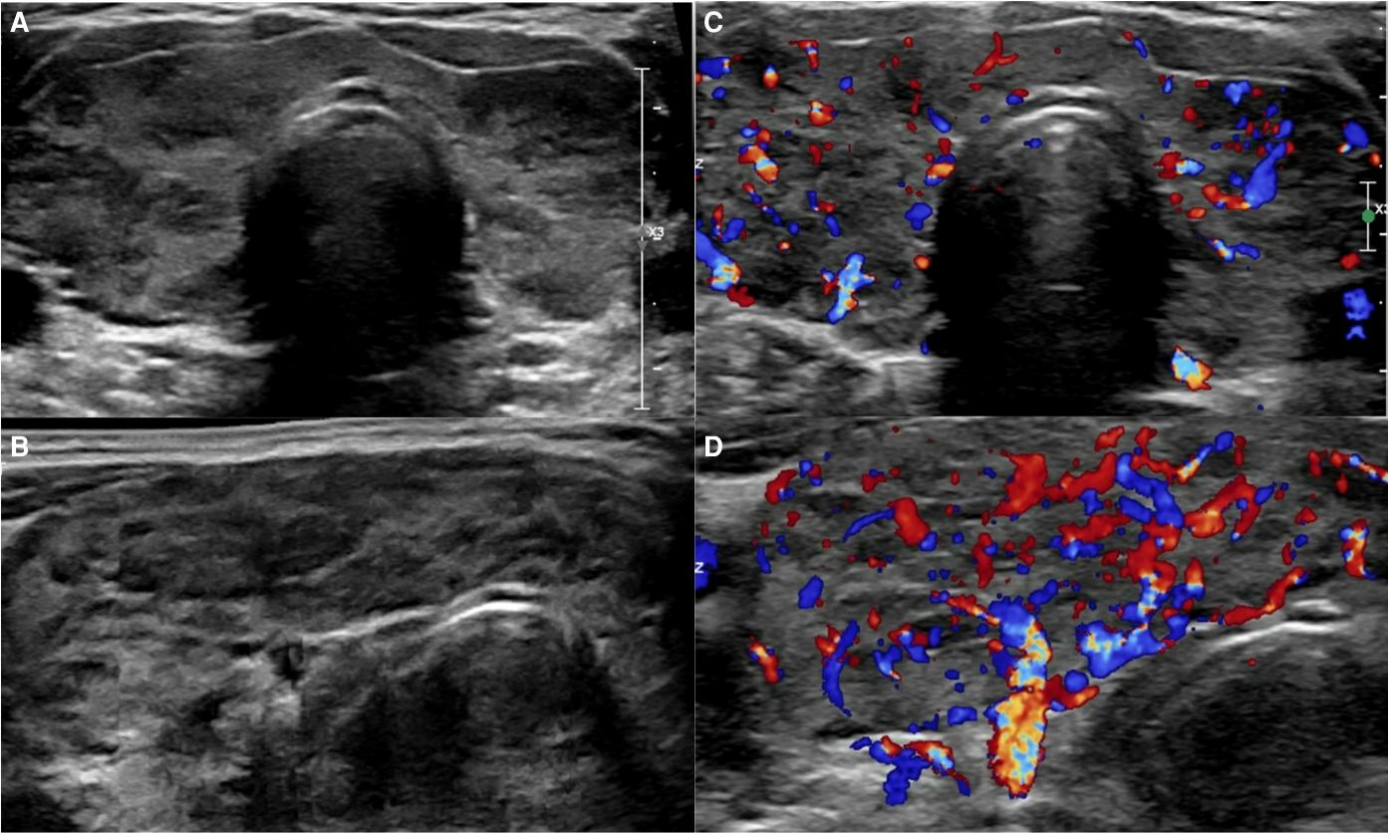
Thyroid ultrasound with color-flow Doppler. (A, C) Gray-scale images show diffuse thyroid enlargement and heterogeneous echotexture. (B, D) Color Doppler images demonstrate diffuse symmetric hypervascularity consistent with GD.
